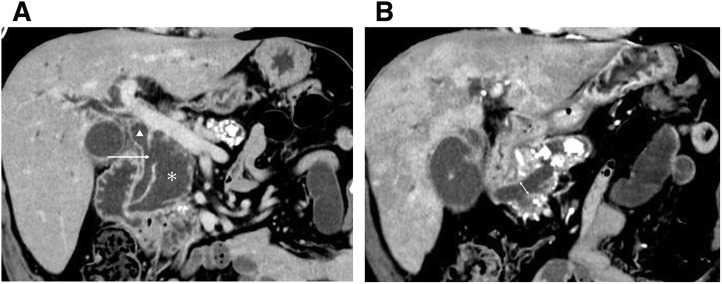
CT image. (A) Contrast-enhanced CT image showing communication between the IPMN and the common bile duct (*; IPMN, ▲; common bile duct, arrow; communication between IPMN and common bile duct). (B) Main pancreas duct enlarged 11 mm at pancreas head. Pancreatic stones are present throughout the pancreas (double-headed arrow; maximum diameter of main pancreas duct). IPMN, intraductal papillary mucinous neoplasm
